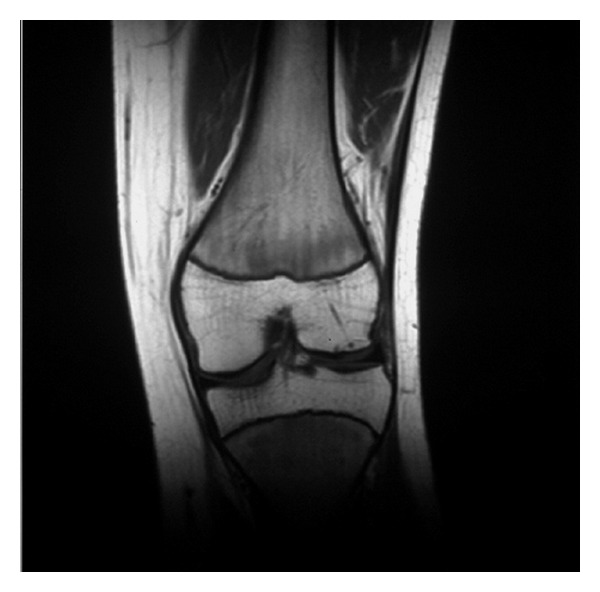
Postoperative MRI image showing consolidation of the osteochondral fragment and biodegradation of the pins in an 11-year-old patient at one-year followup.
